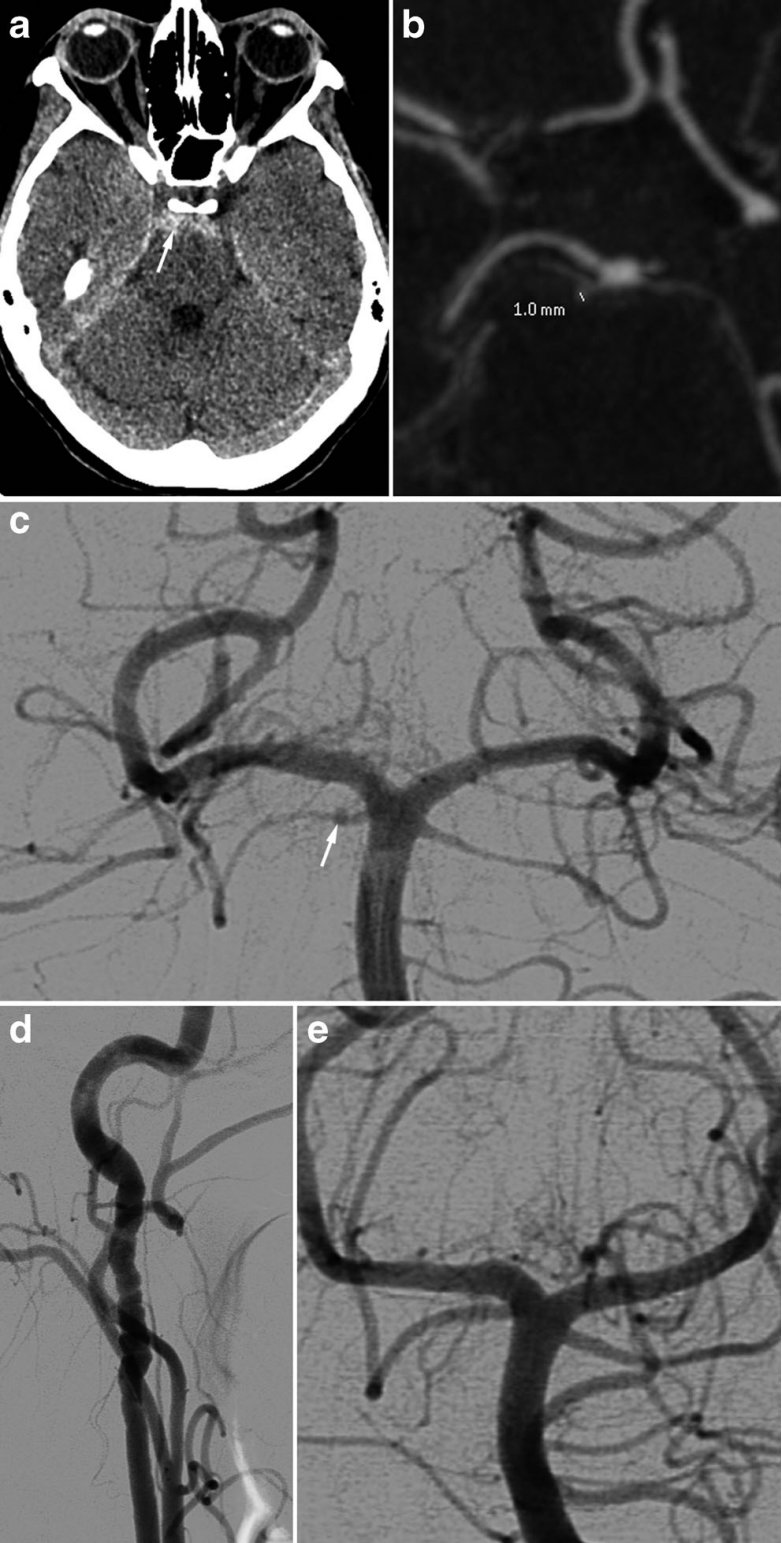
Radiologic findings in a 48-year-old female presenting with posterior headaches. The initial CT shows SAH of the posterior fossa. The angiography found dissection of the right superior cerebellar artery. Study of the supra-aortic arteries shows typical FMD images. Ad integrum restitution at 3 months. a Cerebral non-enhanced CT showing prepontine SAH (*white arrow*). b and c Cerebral CTA and angiogram showing a dissection of the right superior cerebellar artery. d Right carotid angiogram showing showing classical FMD imaging findings. e The 3-month follow-up angiography showing ad integrum restitution of the artery, in favour of arterial dissection
